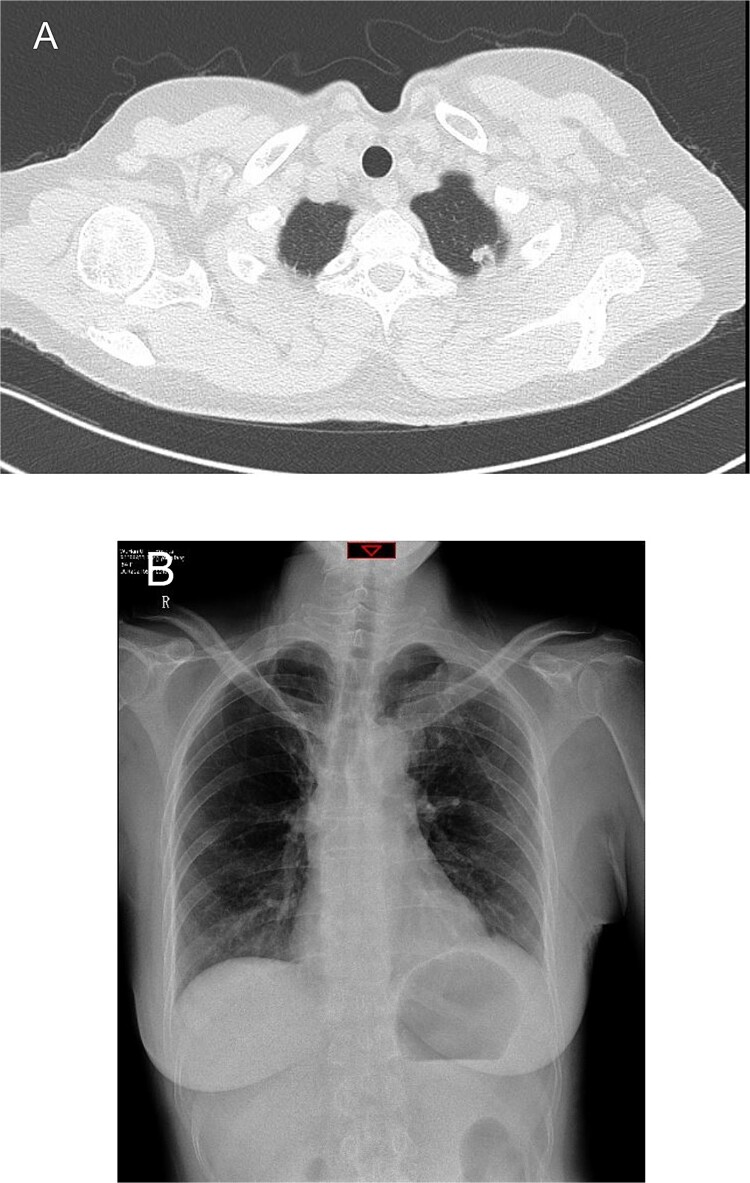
Preoperative chest computed tomography and postoperative day one chest X-ray. (A) Computed tomography shows a solid nodule in the S1+2 segment of the left upper lobe measuring 11 mm × 8 mm. (B) Postoperative day one chest X-ray indicates routine postoperative changes.
